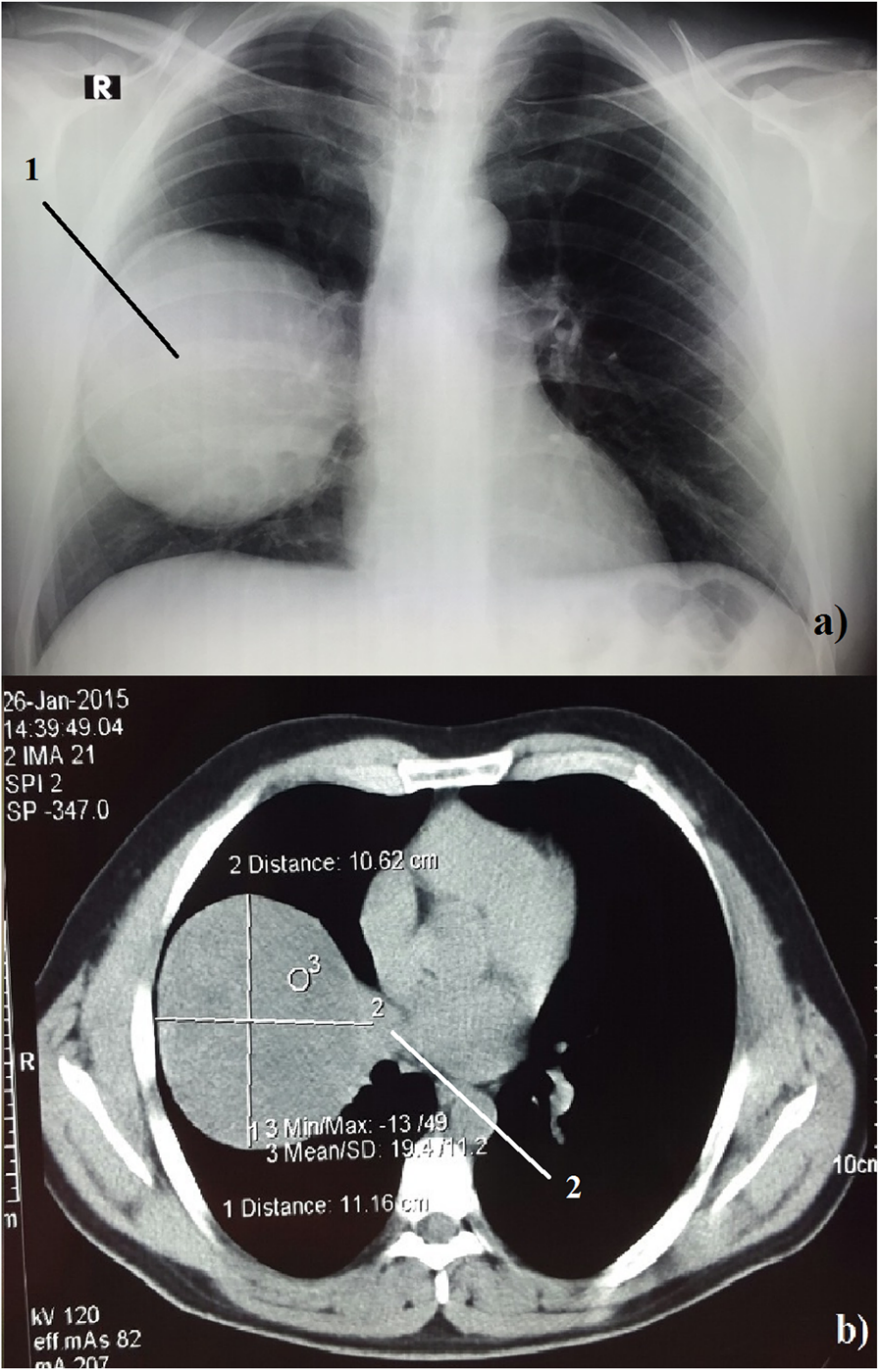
a Patient’s pulmonary X-ray image with a large tumor located in the right lung (10,6x11,1x11,6 cm) (1). b CT-scan image of the patient’s lungs. The tumor is originating from the right main bronchus (2)
